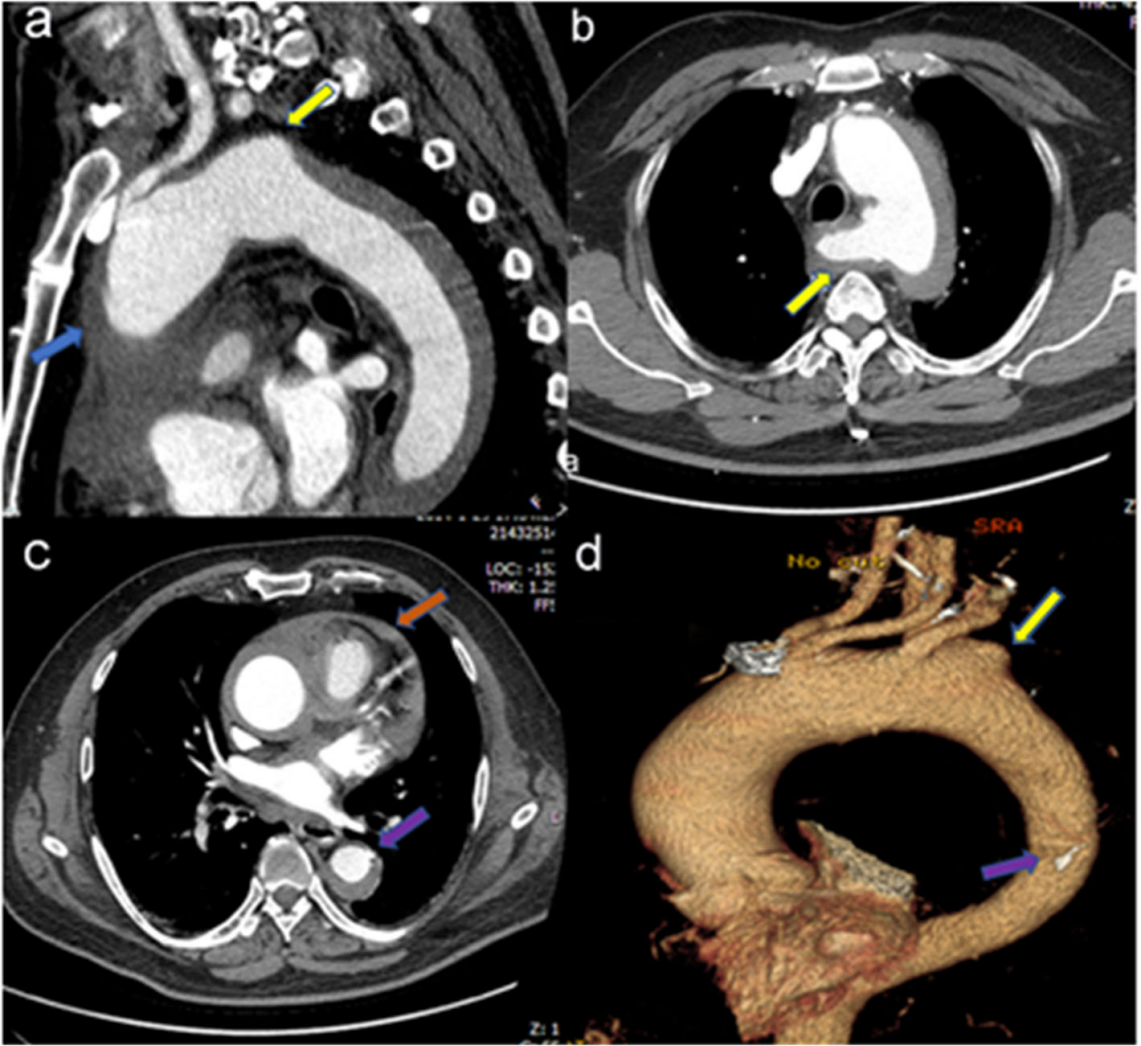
The computed tomography angiography of the patient on admission. a The ascending aortic intramural hematoma (blue arrow) and the Kommerell’s diverticulum (KD, yellow arrow). b KD, (yellow arrow): the aneurysmal aortic dilatation occurs at the origin of the aberrant right subclavian artery (ARSA). c A penetrating atherosclerotic ulcer (PAU, purple arrow) located in descending aorta and pericardial effusion (brown arrow). d PAU (purple arrow) and KD (yellow arrow) at three-dimensions reconstruction
